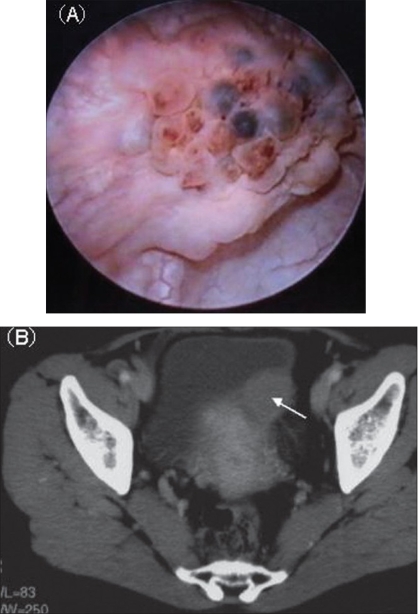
(A) Cystoscopic examination revealed a non-papillary tumor with blueberry spots in the urinary bladder. (B) CT revealed an invasive mass in the bladder
